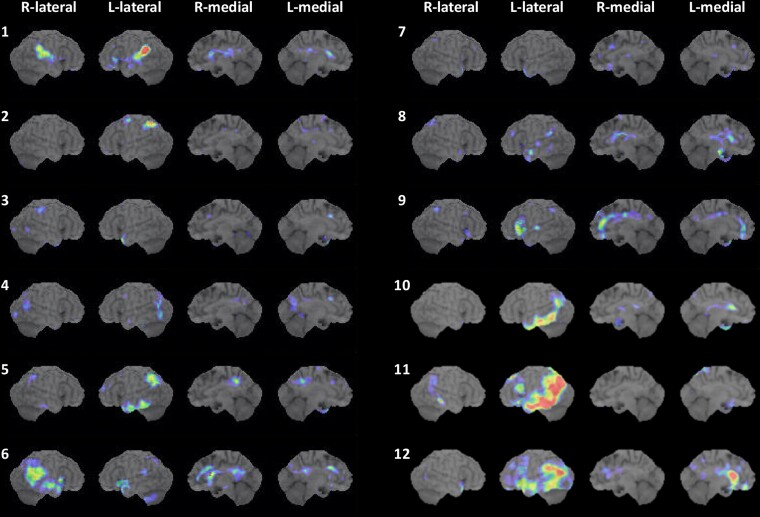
Brain SPECT. Brain SPECT analysed with 3D stereotactic surface projections revealed patterns of significant hypoperfusion in each of the 12 cases with an unclassified fluent variant of primary progressive aphasia. Case numbers are shown to the left of each set of images. Cases 1–8: progressive anomic aphasia; Cases 9–11: progressive TCSA; Case 12: progressive Wernicke’s aphasia. SPECT, single-photon emission computed tomography; L, left; R, right; TCSA, transcortical sensory aphasia.
